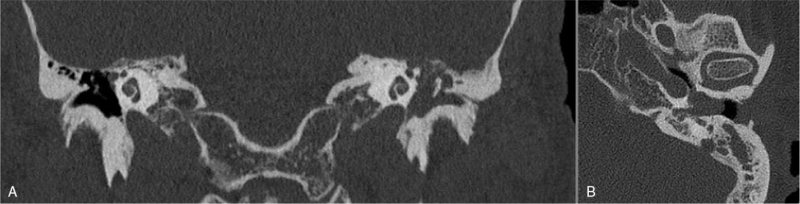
Computed tomography (coronal [*A*] and axial planes [*B*]) revealed soft tissue invasion of the left mastoid bone and the middle ear cavity, completely embedding the ossicular chain.
